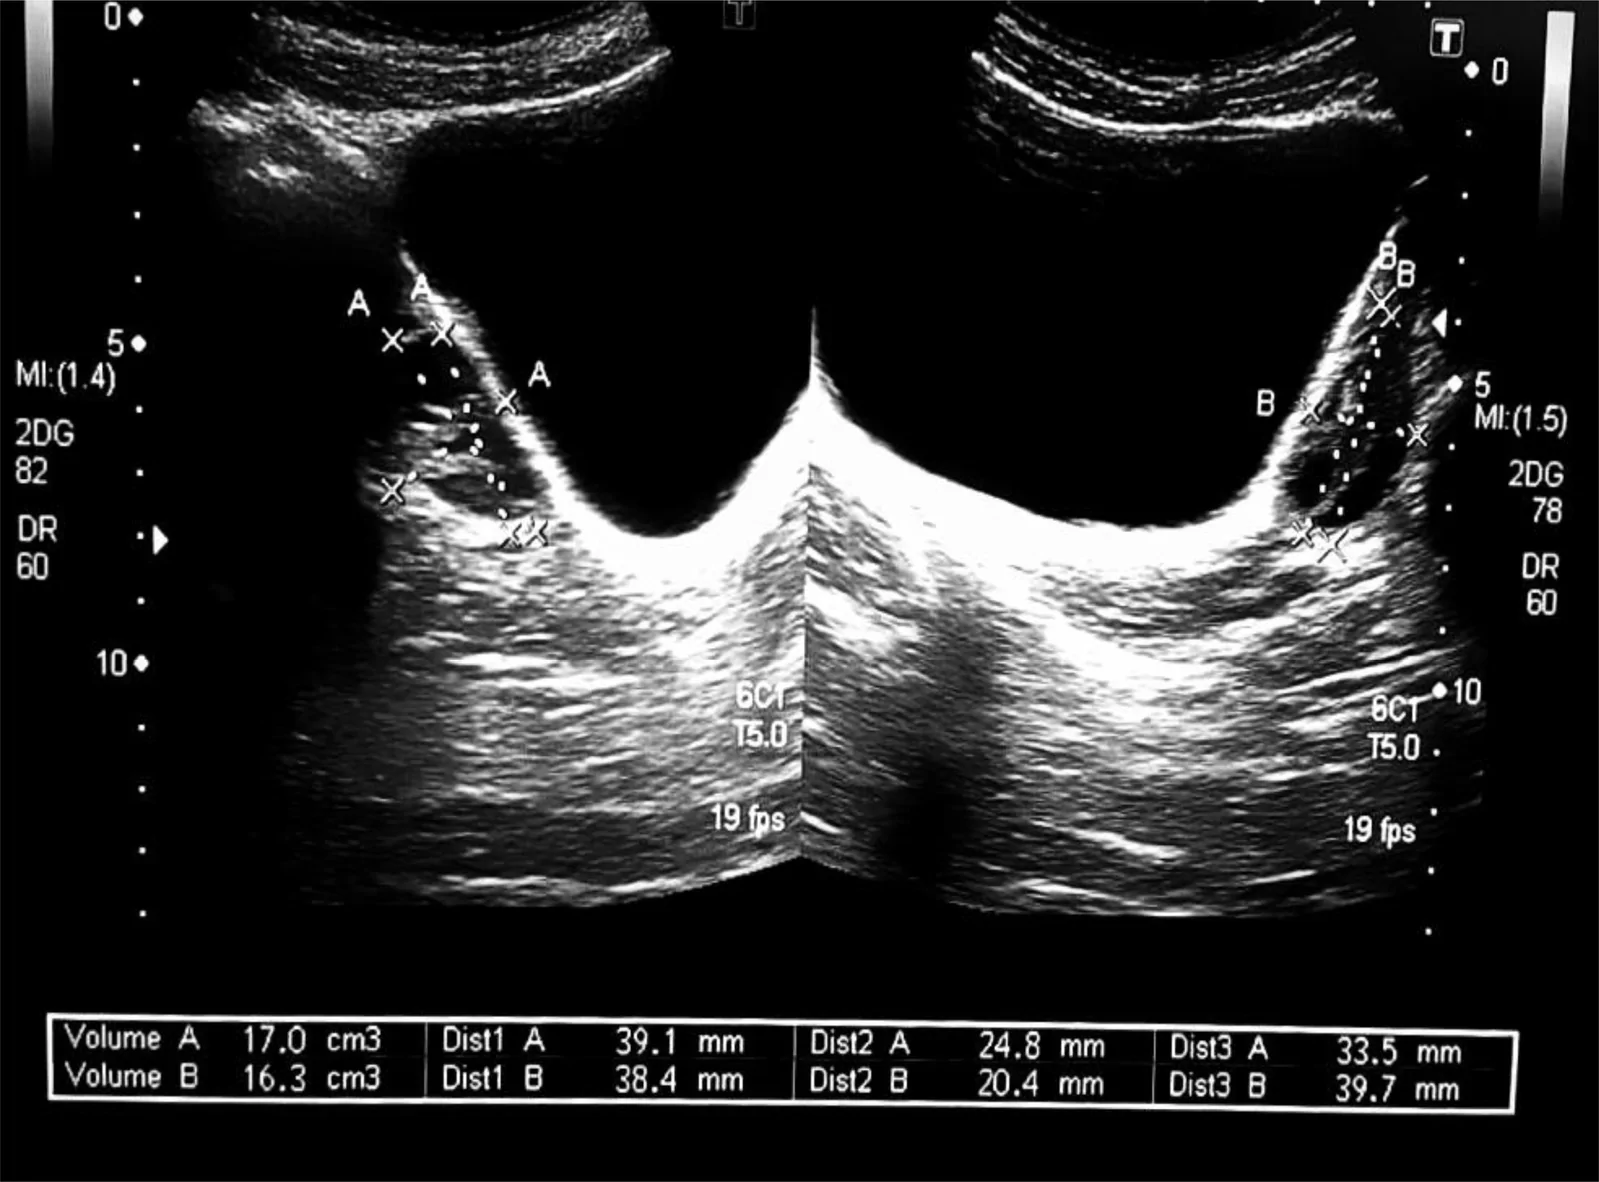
Ultrasound image of bilateral PCOS in a 22-year-old woman with moderate ovarian volume.
